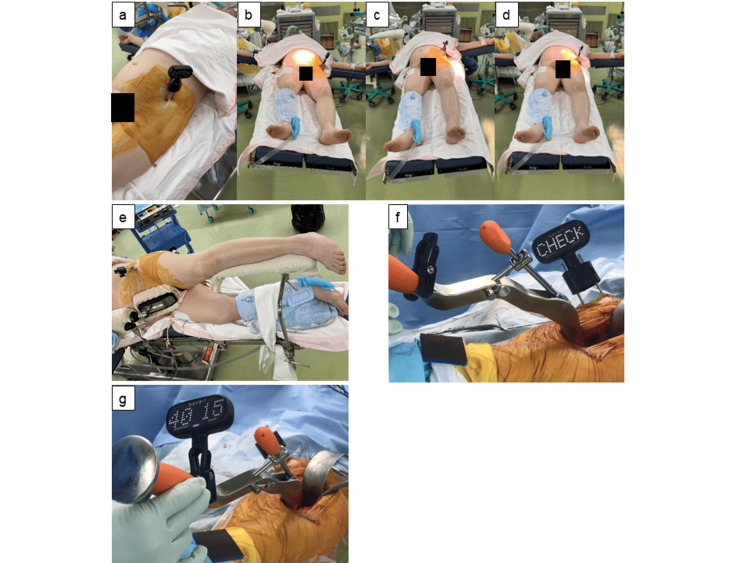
NAVBIT, a newly available portable navigation system. Insert two navigation pins parallel to the iliac crest and attach the NAVBIT sensor (a). Table tilt registration. Tilt the bed 10° to the left and register (b), tilt the bed 10° to the right and register (c), and return the bed to the horizontal position and register (d). After registration in the supine position, change the patient’s position to the lateral position and perform surgery in the lateral position (the flip technique) (e). Press fit the cup with a radiographic inclination of 40° and radiographic anteversion of 15° (f, g).
